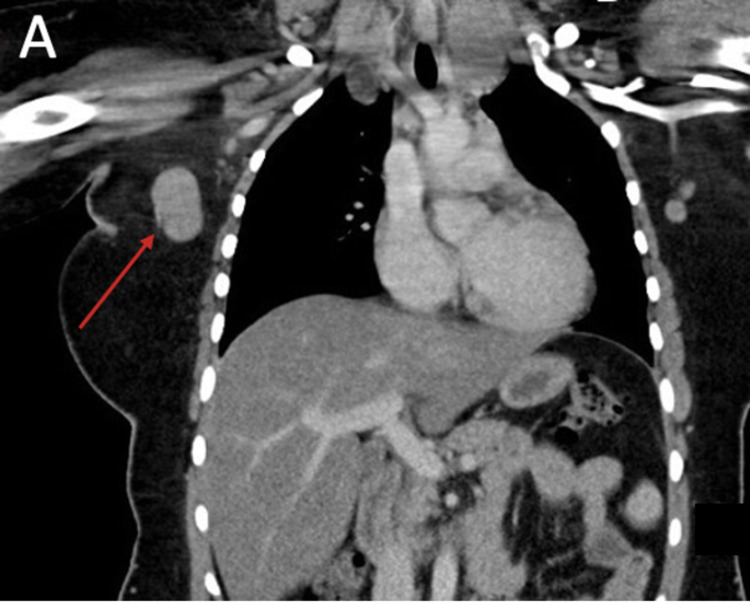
Coronal cut of the CT scan showing enlarged right axillary lymph node.
